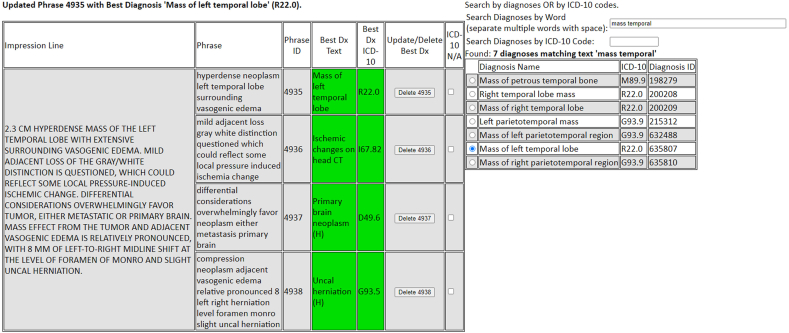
Example of the user interface used for “manual coding”; in this patient, a noncontrast CT report's Impression section (note: patients' identifiers swapped with alias data) describes a left temporal lobe mass. The user's entered search for “temporal mass” yielded several possible codes (*right*), and then the user selected “R22.0” (‘mass of left temporal lobe’) for this finding (*middle, in green*). Three other such searches for phrases by the user yielded codes for “ischemic changes on head CT” (I67.82), “primary brain neoplasm” (D49.6), and “uncal herniation” (G93.5), which are highlighted in *green* as well; the user subsequently attached these 4 manually-selected codes to the report. (For interpretation of the references to colour in this figure legend, the reader is referred to the Web version of this article.)
